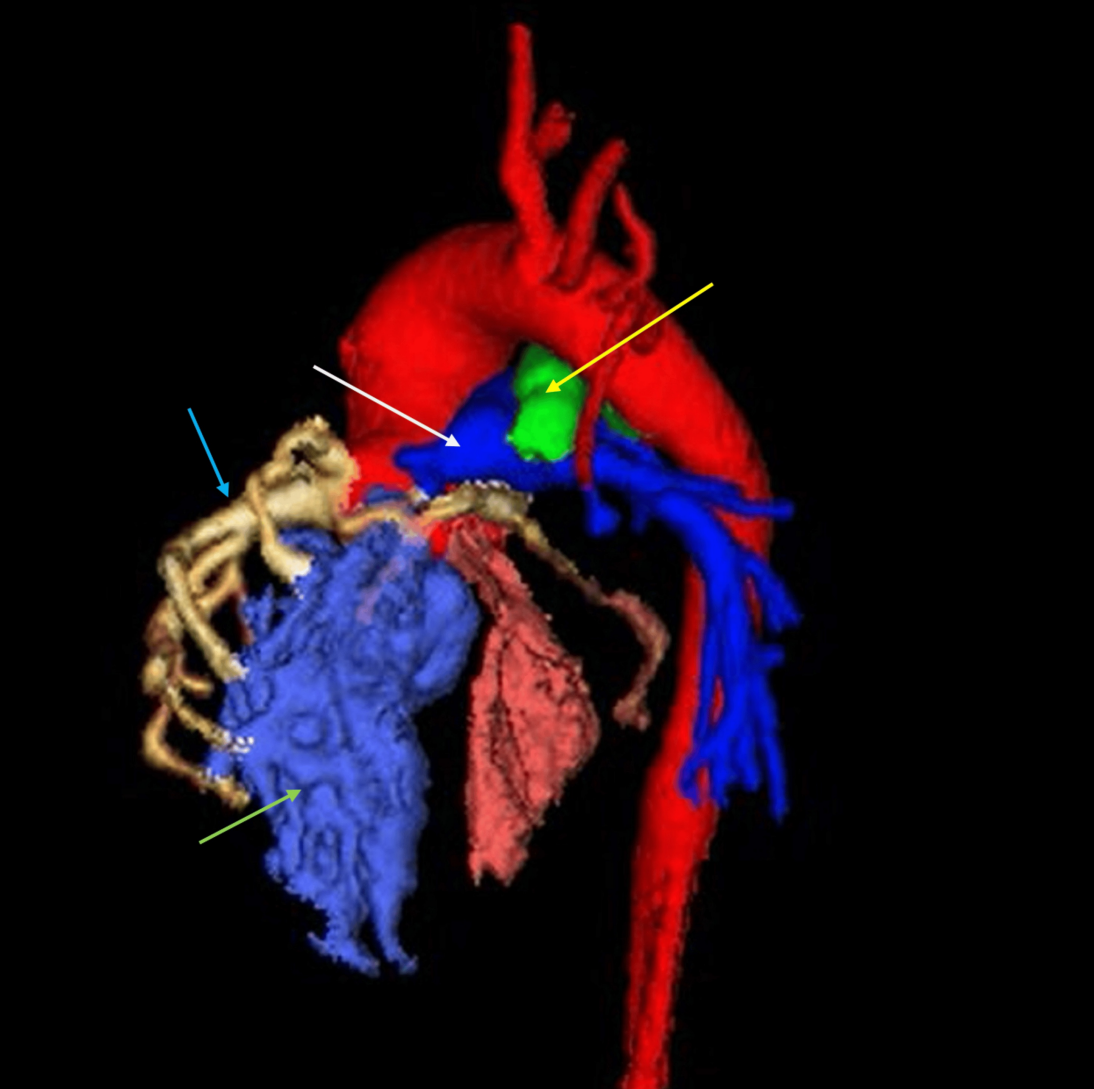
Three-dimensional reconstruction of a computed tomography image demonstrating markedly enlarged coronary arteries (blue arrow) communicating with the hypertrophied and hypoplastic right ventricle (green arrow), complete atresia of the pulmonary artery (white arrow), and an enlarged, tortuous patent ductus arteriosus (yellow arrow).
